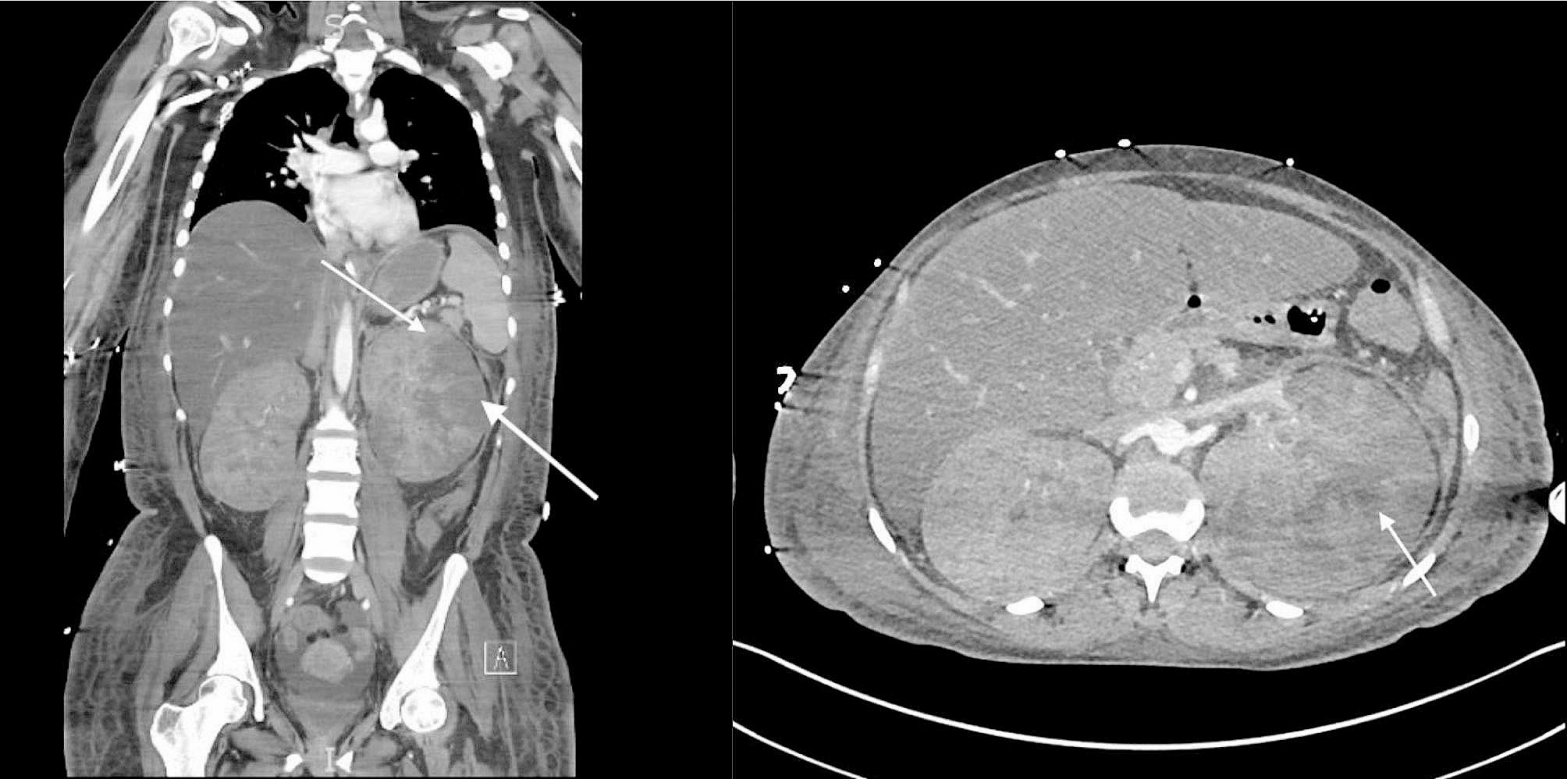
Computed tomography scan of the abdomen/pelvis with intravenous contrast. Massive nephromegaly is present with areas of hypoperfusion and/or infarct (arrows)
